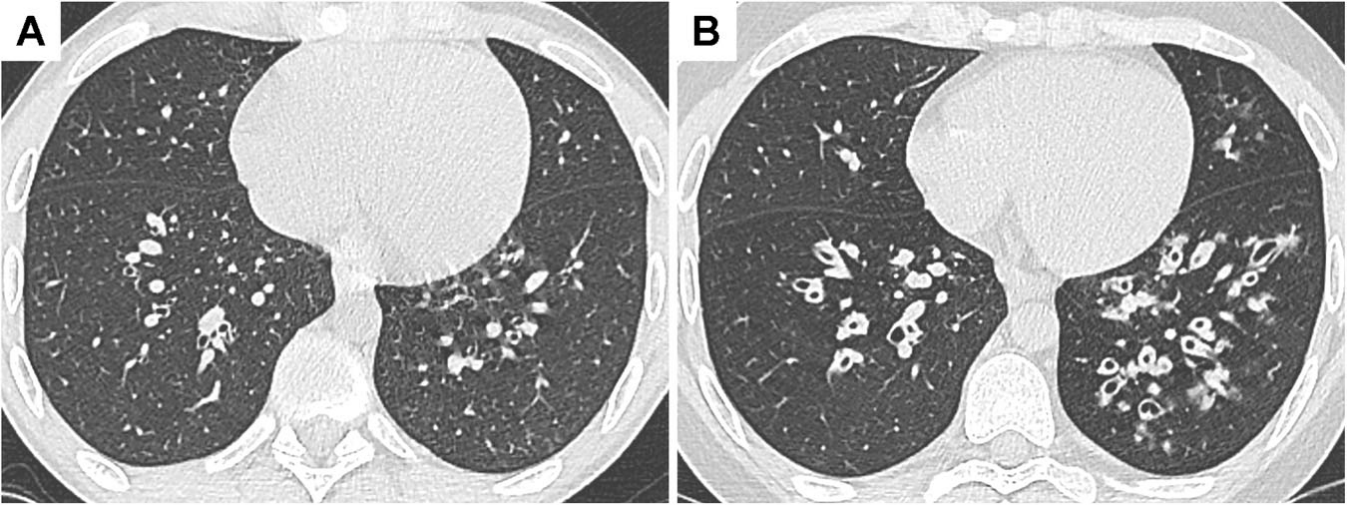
Airway disease in a patient with severe Crohn’s disease. A 18-year-old developed a cough a few months after colectomy. A chest CT scan performed at that time showed only slight bronchial wall thickening in the lower lobes. B Seven years later, chest CT shows bilateral bronchiectasis in the lower lobes with severe bronchial wall thickening
